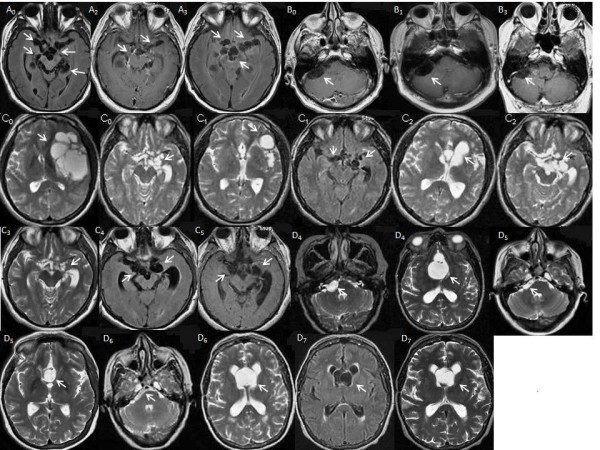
MRI of patients. Letters correspond to each of the patients and numbers corresponds to the moment of the MRI in relation to the number of cysticidal cycles administered (0: basal MRI, 1: after the first cycle, etc.). A0 (MRI T2 FLAIR): vesicular cysticerci in basal cisterns. Decrease (A2, MRI CE, T1) and increase (A3, MRI T2 FLAIR) in parasites. B0 (MRI CE T1): Vesicular parasites in the pontocerebellar angle. B1: Increase of parasite. B3: Persistence of parasite. C0 (MRI T2): Cysticercus in the Sylvian and basal cisterns. C1 (MRI T2, FLAIR): Decrease in Sylvian and persistence of basal cisterns parasites. C2 (MRI T2): Increase of lamina terminalis and basal cisterns parasites. C3 (MRI T2): Decrease of basal cisterns parasites. C4 (MRI CE T1): Increase of basal cisterns parasites and appearance of new ones. C5 (MRI FLAIR): Persistence of parasites. D4 (MRI T2): Parasites in the right pontocerebellar angle and in the lamina terminalis cisterns. D5 (MRI T2): Decrease of parasite volume. D6 (MRI T2): Increase of the lamina terminal cistern vesicles. D7 (MRI FLAIR, T2): Persistence of vesicles.
